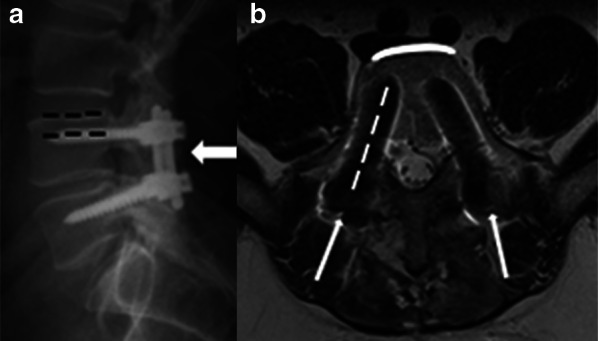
A typical arthrodesis with rod and transpedicular screws—posterior approach for degenerative disc disease. Lateral radiography (a) and T2W axial MR image (b) after a fusion procedure using stabilization hardware consisting of bilateral rods and transpedicular screws at the L4-5 intervertebral level (arrow). There is optimal pedicle screw positioning. The screws traverse the pedicle without lateral or medial angulation (thin arrow and white dashed line) and the anterior vertebral body cortex is intact (curved line). The screws are also parallel to the vertebral body endplate in the lateral/sagittal plane (black dashed line)
